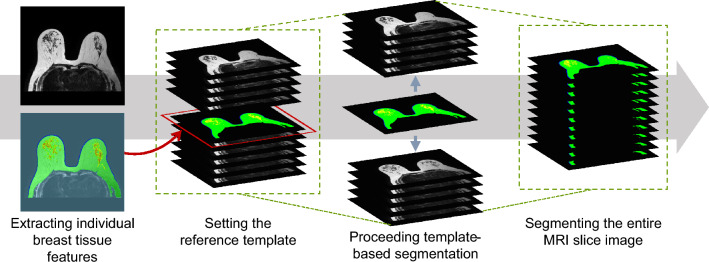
Segmentation of full MRI slice images through template-based segmentation.
